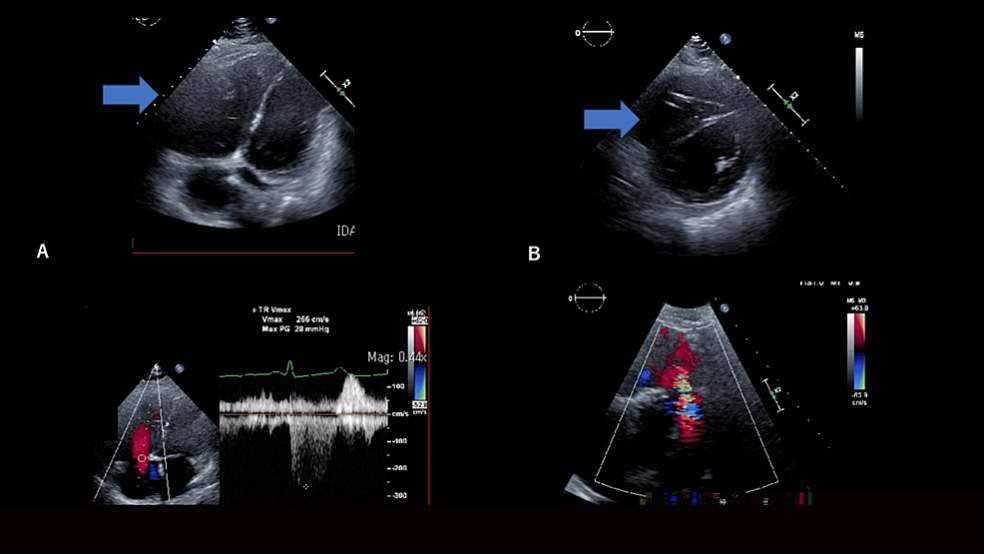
Echocardiography on the seventh day of admission The ejection fraction (EF) was 61.1%. A: Apex four-chamber view. The right ventricle is enlarged (blue arrow). B: Short axis view. The right ventricle is compressing the septum (blue arrow). C: Moderate TR is observed. D: Severe PR is observed. Pressure half-time (PHT) was 83 msec.
